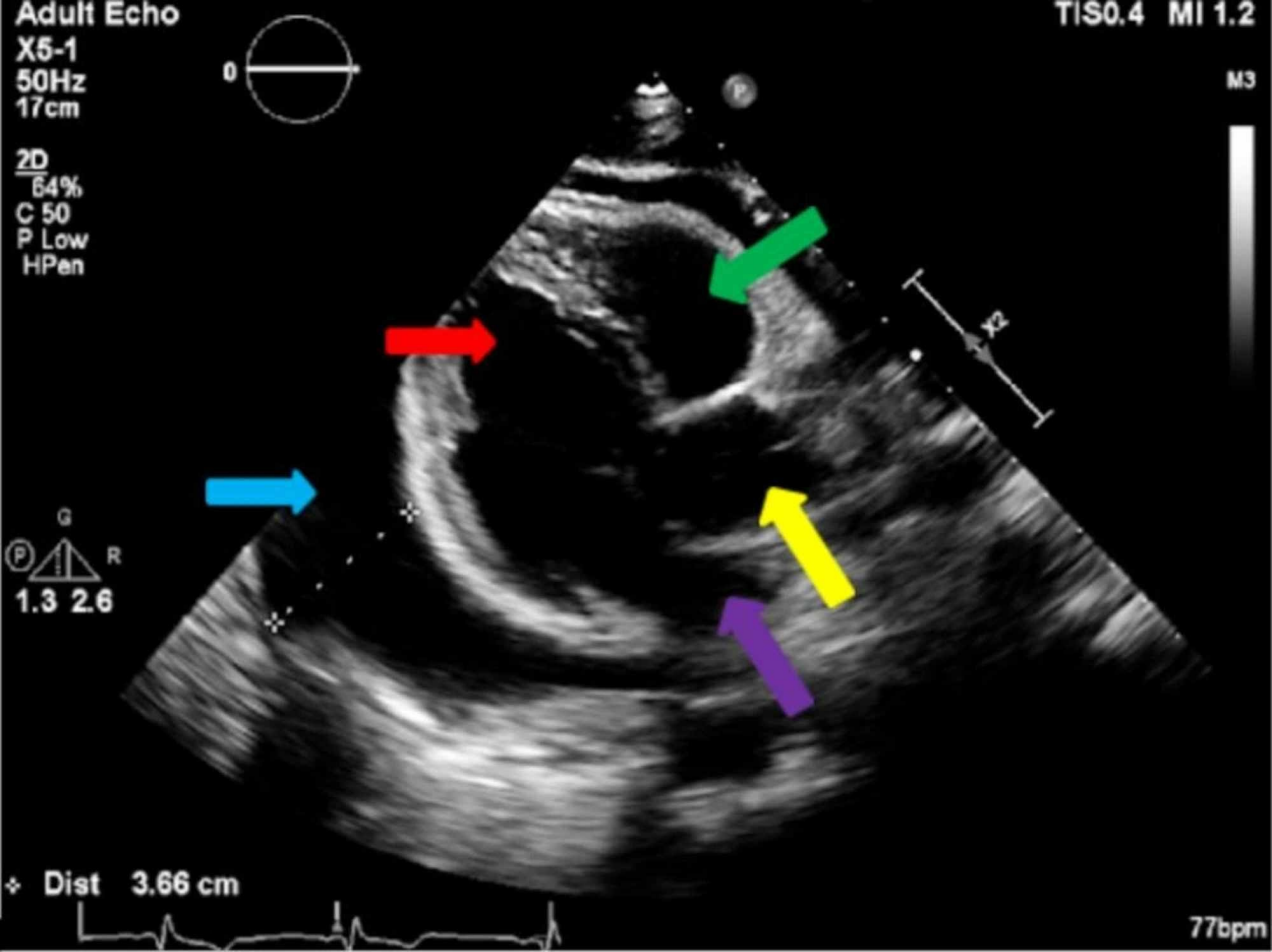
Parasternal long axis view of a large pericardial effusion measuring 3.66 cm (blue arrow), left ventricle (red arrow), compressed right ventricle (green arrow), aorta (yellow arrow), and the left atrium (purple arrow).
